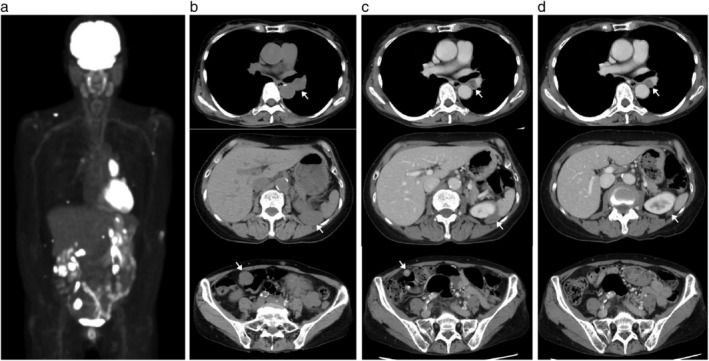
PET‐CT and follow‐up CT images. Arrows indicate tumors. (a) Pretreatment positron emission tomography (PET)‐computed tomography (CT) scan of the patient. (b) Pretreatment CT scan. (c) CT scan after one dose of pembrolizumab. (d) CT scan after eight doses of pembrolizumab.
